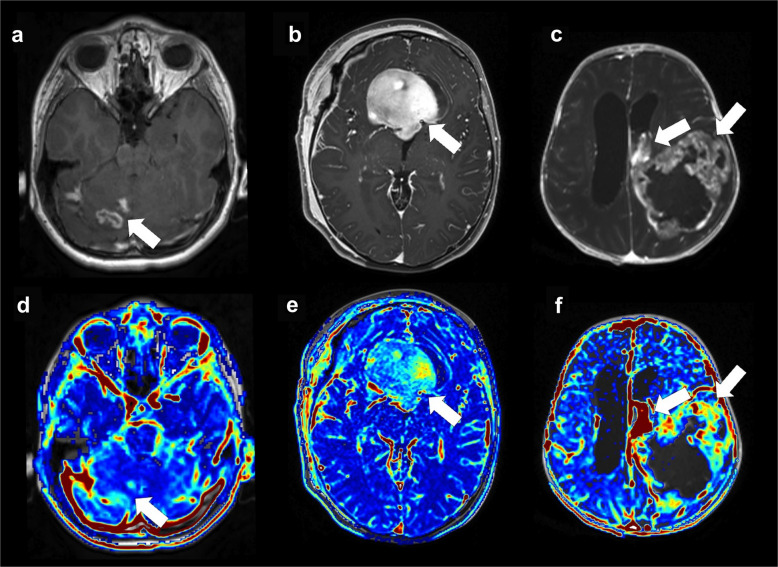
Axial post-contrast T1-weighted structural (a-c) and dynamic contrast-enhanced (d-f) magnetic resonance images show brain tumours in three children. a, d A 12-year-old boy with a rosette-forming glioneuronal tumour (World Health Organization (WHO) grade 1). b, e A 5-year-old girl with pilocytic astrocytoma, *KIAA1549::BRAF* fusion-positive (WHO grade 1). c, f A 1-month-old girl with a supratentorial ependymoma, *YAP1*-fusion-positive (WHO grade 3)
